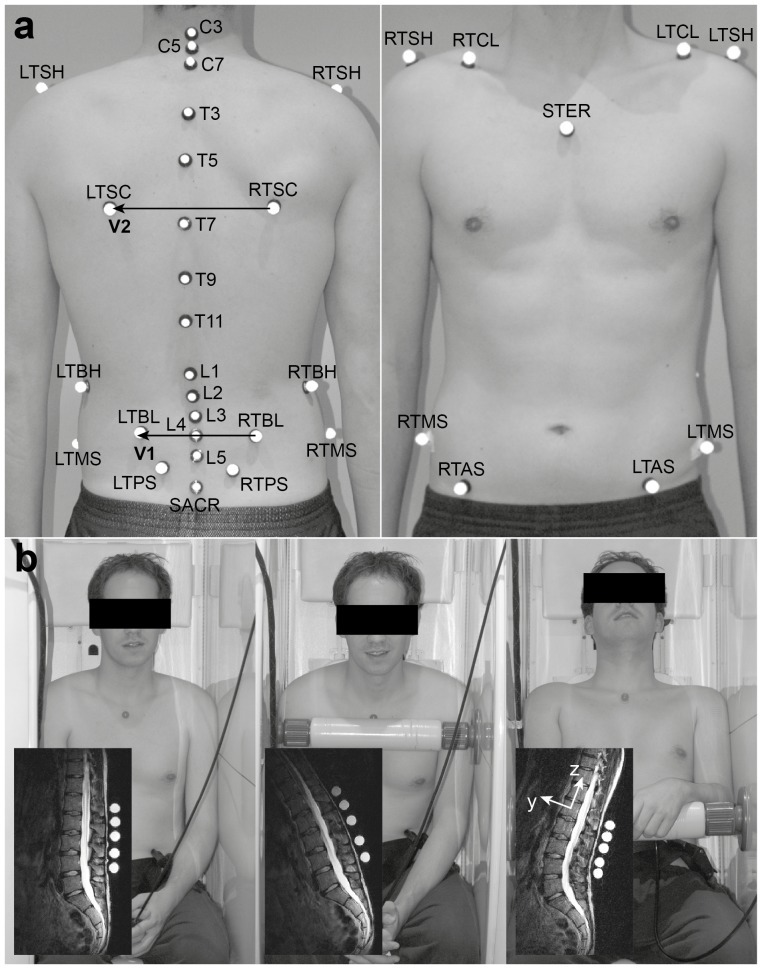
Measurement set-up including (a) the “IfB-marker-set” of the trunk and the pelvis (for explanation of abbreviations and for segmental allocation, see Table 2) and (b) the three analysed seating positions with an example of a corresponding MR image including a local coordinate system of a vertebral body. Upright seating position: lower spine had partial contact with the backrest, and the whole upper body was in an upright position. Flexed seating position: upper body was tilted about 30° forward and supported on a bar, while the arms were rested on their lap. Extended seating position: the subject's bottom was pushed approximately 20 cm forward and the head was supported by the backrest. (from left to right).
